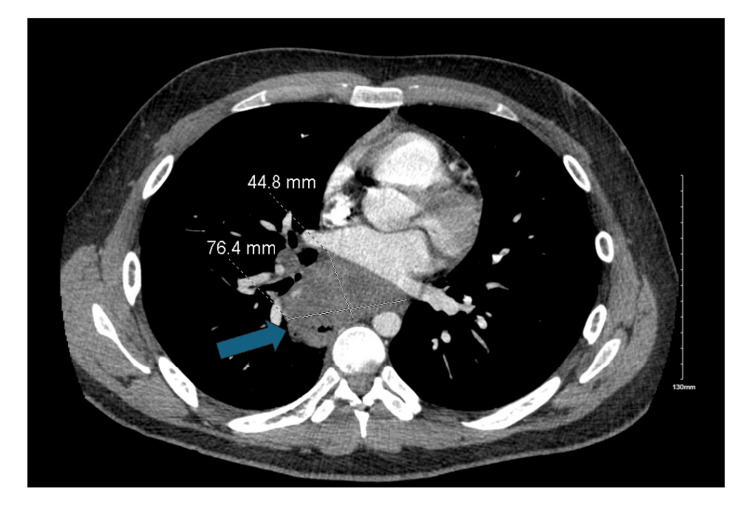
CT thorax on admission CT thorax with contrast on admission demonstrating a 7.6 cm necrotic posterior mediastinal mass partially encasing the right inferior pulmonary vein.
